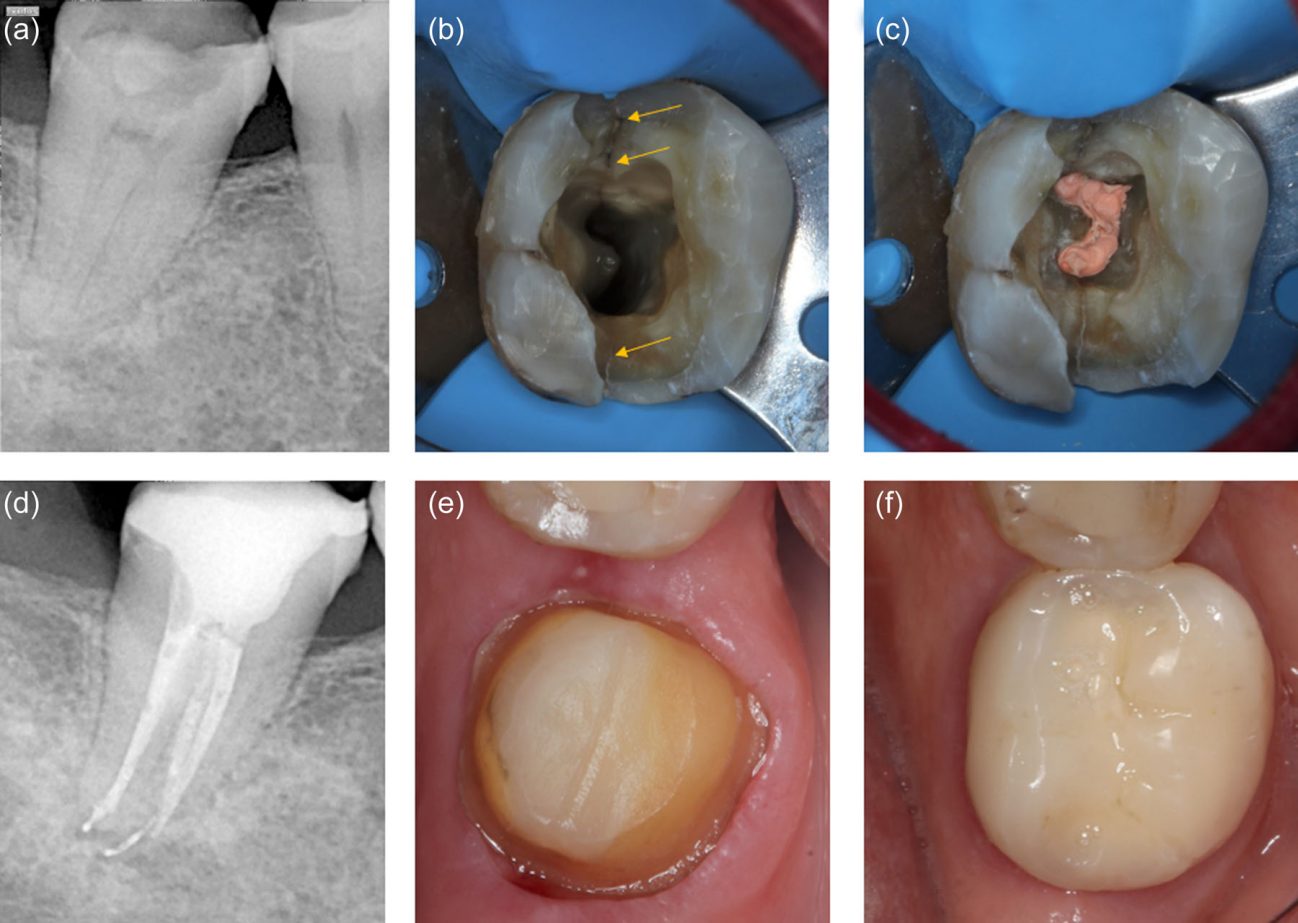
Treatment sequence of a cracked 46 with irreversible pulpitis (a) preoperative long‐cone periapical radiograph (b) access cavity for endodontic treatment revealing mesial and distal crack lines (arrows) and C‐shaped canal configuration (c) root canal obturation completed (d) periapical radiograph after completion of endodontic treatment (e) crown preparation (f) occlusal view after cementation of a metal ceramic crown.
